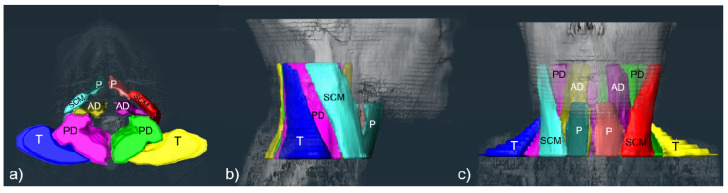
Three-dimensional reconstruction of MRI muscle volume segmentations (case 41) in (a) axial, (b) right sagittal, and (c) coronal views: trapezoid muscle (“T”, right side blue, left side yellow), posterior deep muscles (“PD”, right side pink, left side green), anterior deep muscles (“AD”, right side dark yellow, left side purple), sternocleidomastoid muscle (“SCM”, right side light turquoise, left side red), and pretracheal muscles (“P”, right side dark turquoise, left side rose).
